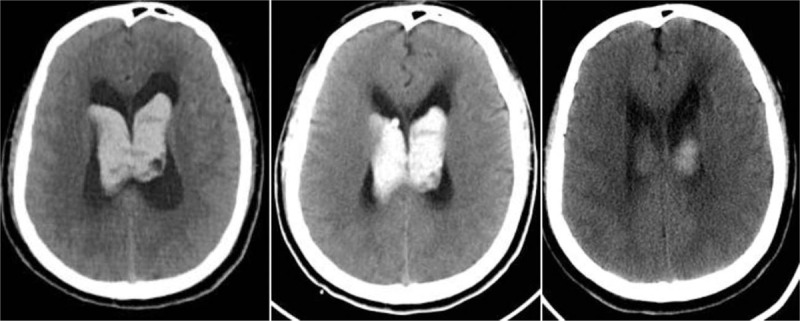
CT scan of an IPIVH patient at admission, 1 day after EVD and at discharge. CT = computed tomography, EVD = external ventricular drainage, IPIVH = idiopathic primary intraventricular hemorrhage.
